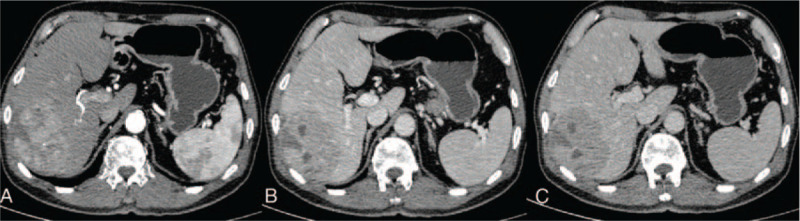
Total abdominal 3-phase enhanced CT imaging of the patient (gender: male, age: 77 yr) suffering from PC accompanied by primary HCC. (A) In the arterial phase, the lesions look unevenly enhanced and the blood supply artery is visible. (B and C) The venous phase and the equilibrium phase, the degree of lesion enhancement is reduced with some lipid components, suggesting that the lesion was derived from liver cells. CT = computed tomography, HCC: hepatocellular carcinoma, PC: prostate cancer.
